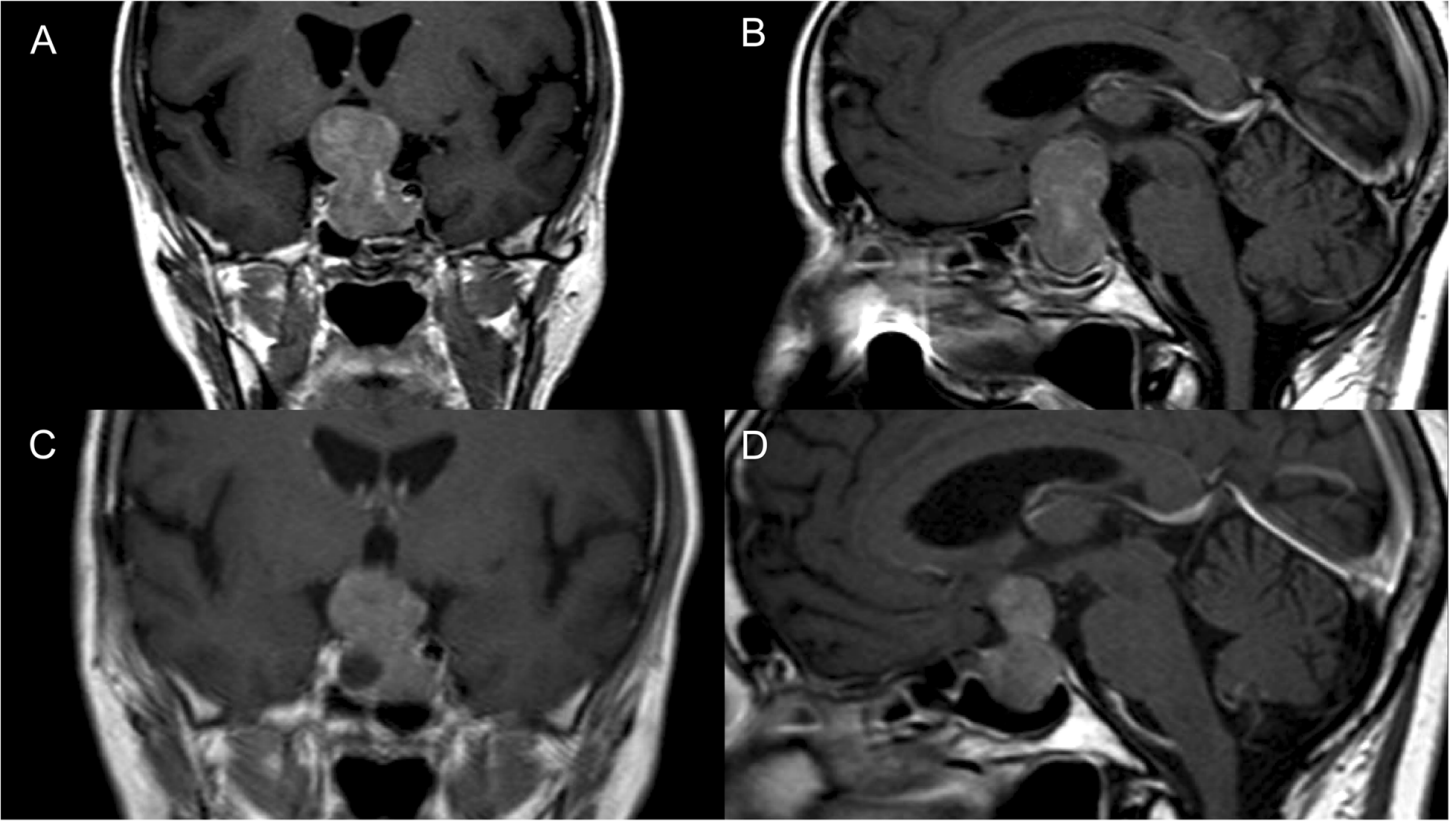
The coronal (a) and sagittal (b) magnetic resonance images of pituitary macroadenoma with suprasellar extension into the left cavernous sinus (Knosp grade 2). The images were obtained before the first surgery in July 2010. The coronal (c) and sagittal (d) magnetic resonance images of pituitary macroadenoma with prolonged tumour growth with essential suprasellar and extension into both cavernous sinuses. The images were obtained before the second surgery in July 2012
